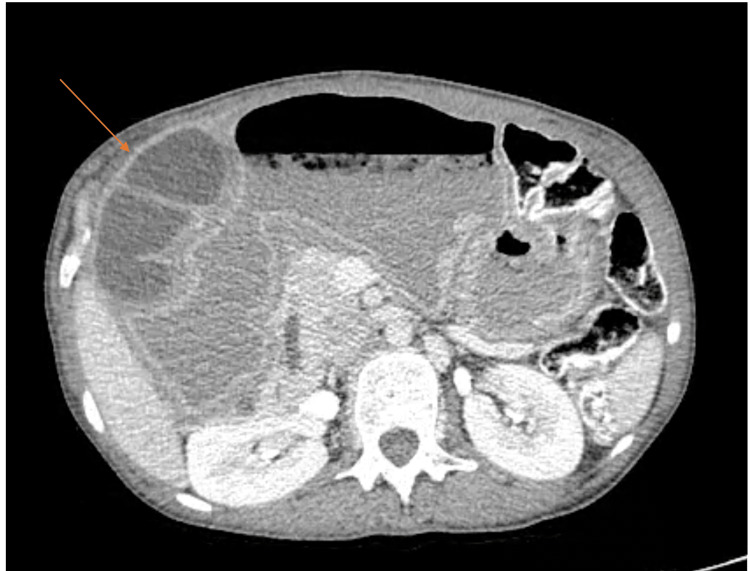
CT scan findings - dilation of the stomach and an associated mass, probably diverticulum, that compressed the stomach.
